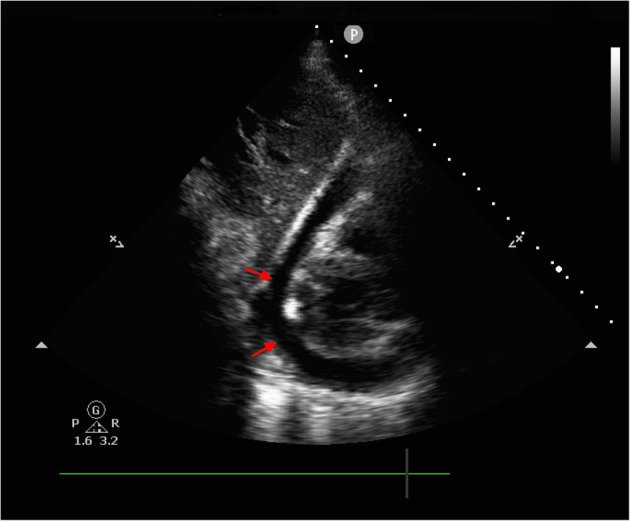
Bedside transthoracic echocardiography revealed massive pericardial effusion (red arrows: pericardial fluid sonolucent area).
